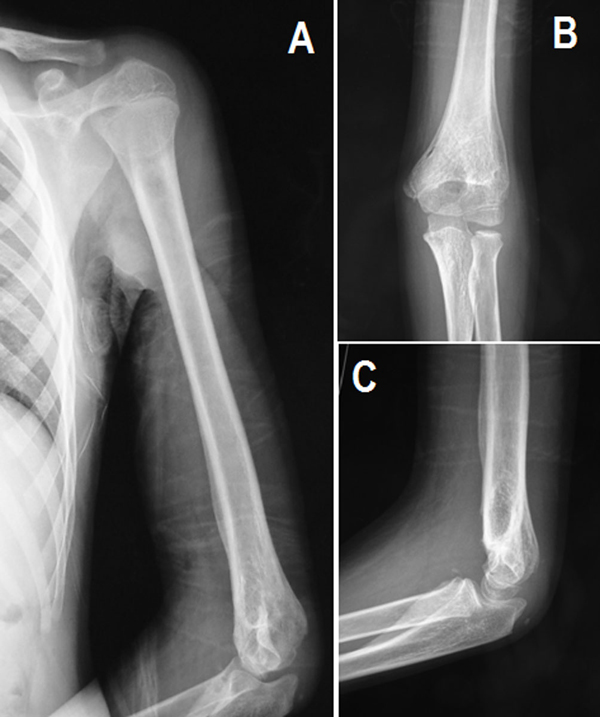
Postoperative roentgenograms of (A) left upper extremity and (B, C) left elbow at six months.
